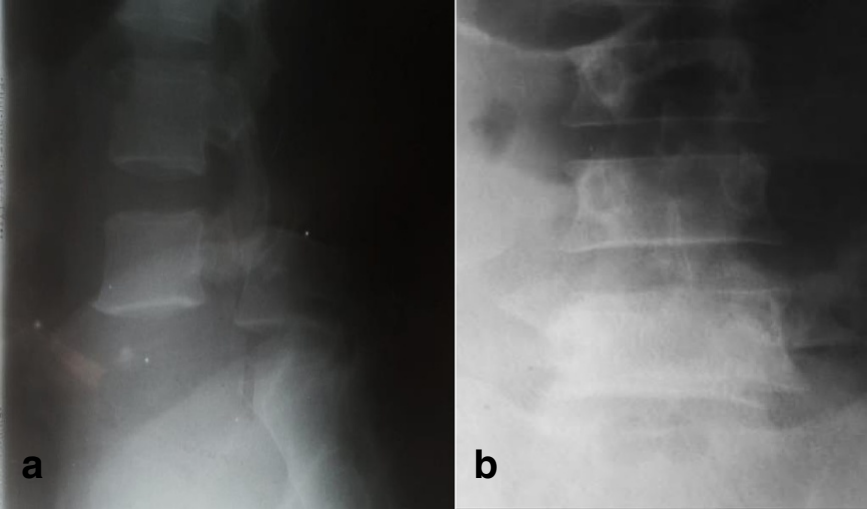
Preoperative radiographs. Plain anteroposterior (a) and lateral radiographs (b) of the lumbar spine showing spondyloptosis with L4 lying anterior to the L5 vertebral body in the sagittal plane.
